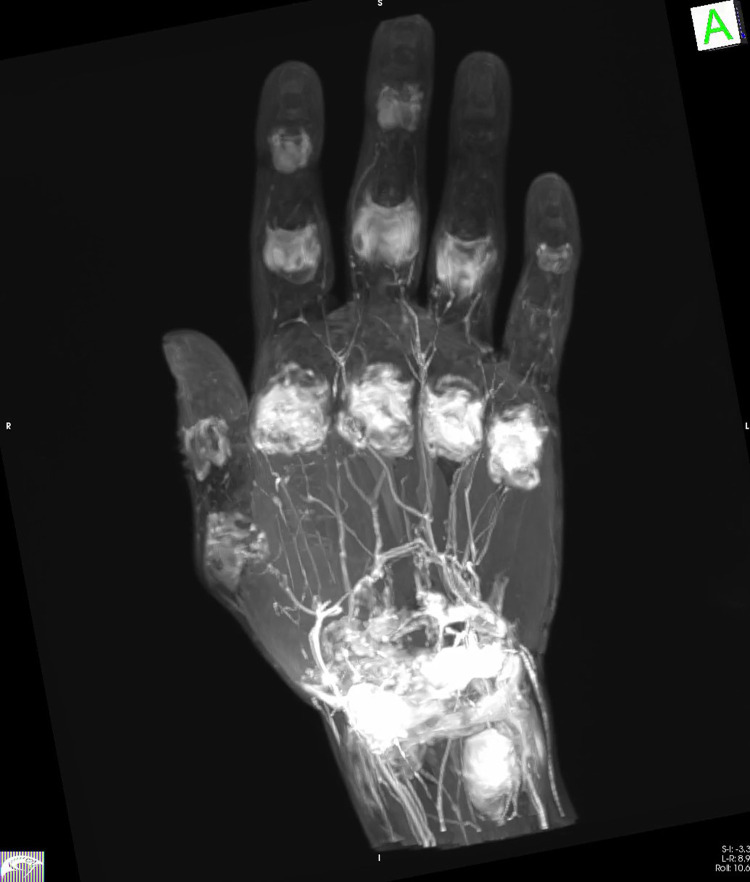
Maximum intensity projection of a magnetic resonance imaging with contrast agent gadobutrol of the patient’s right hand. The video clearly shows all but 1 joint affected by synovial inflammation including distal interphalangeal joint involvement.
